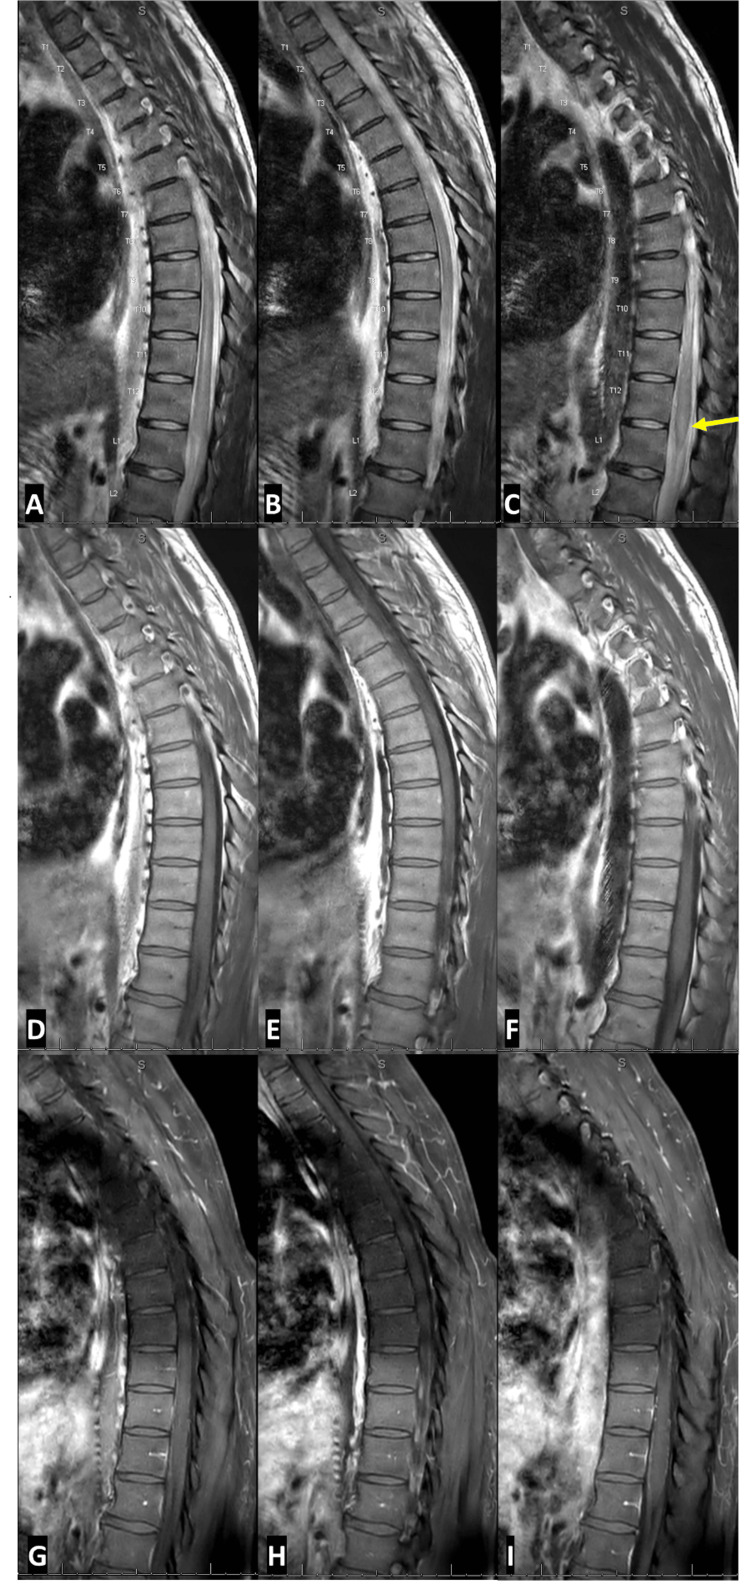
Sagittal T2 (A-C), T1 pre- (D-F), and post-contrast (G-I) MRI thoracic spine from day 2 of hospitalization. The imaging shows edema in the spinal cord extending from T10 to L1 with diffuse enlargement of the cord contour at T11 to L1 (yellow arrow) and without enhancement, suggestive of longitudinally extensive transverse myelitis or LETM.
